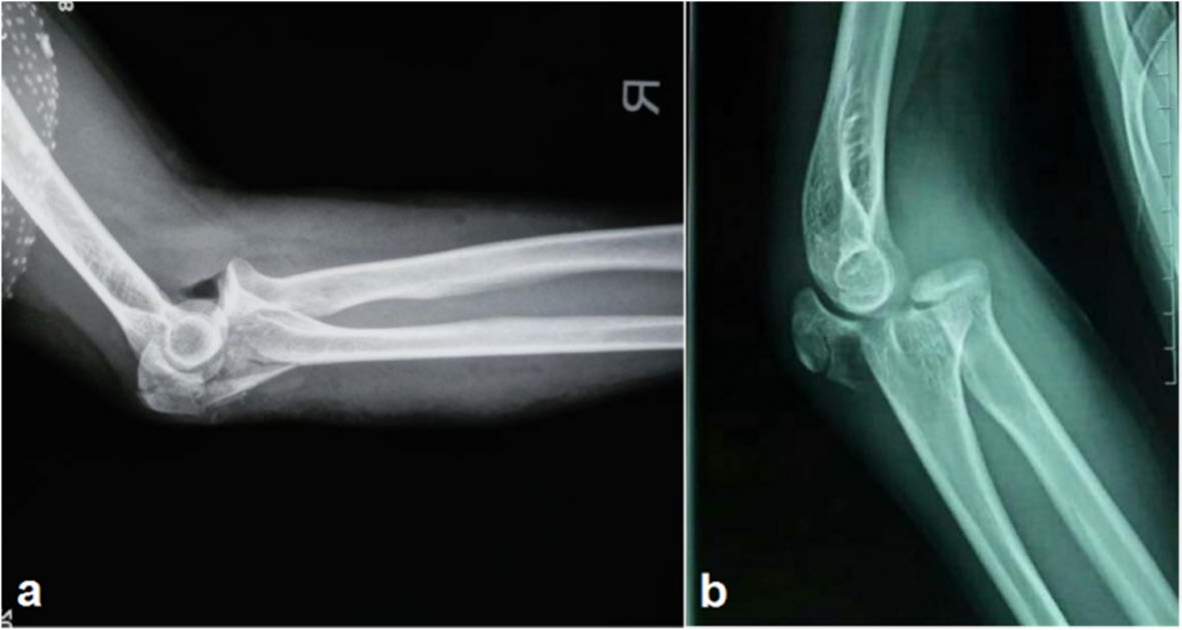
Lateral radiograph of the elbow in a 42-year-old-male patient with comminuted fracture of proximal ulna, combined with fracture of coronoid process and anterior dislocation of distal complex of fracture (a); Lateral radiograph of the elbow in a 14-year-old boy (case 4) with transverse fracture of olecranon, small proximal fracture fragment, combined with anterior dislocation of distal complex of fracture, no involvement of coronoid process (b)
